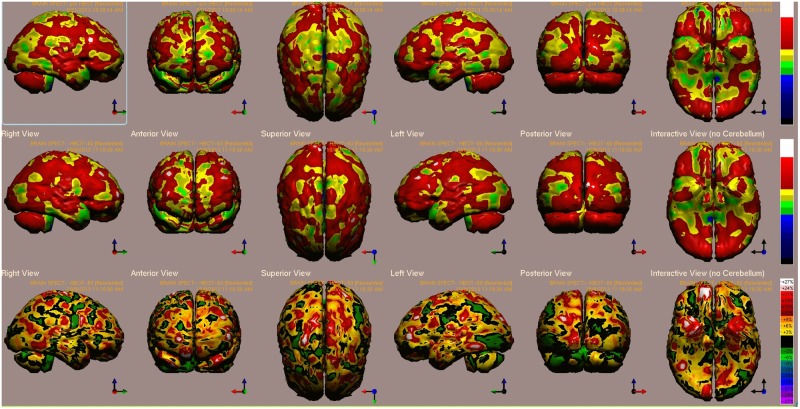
Case 1 single photon emission CT (SPECT) calculated change after hyperbaric oxygen therapy (HBOT) compared to baseline. In the Top two rows colors, represent maximal functional brain activity relative to the entire brain activity; in the Bottom row, colors represent regional changes in functional brain activity. White and red areas show the biggest relative changes in cerebral blood flow/perfusion. Significant changes can be seen at the right prefrontal and temporal areas (white). Abbreviations: Analysis of brain SPECT performed pre- and post HBOT showed the highest relative increase in activity (>15%) in the visual cortex (BA 19), prefrontal cortex (BA 9), orbito-frontal gyrus (BA 11), and paratemporal cortex (BA 37).
